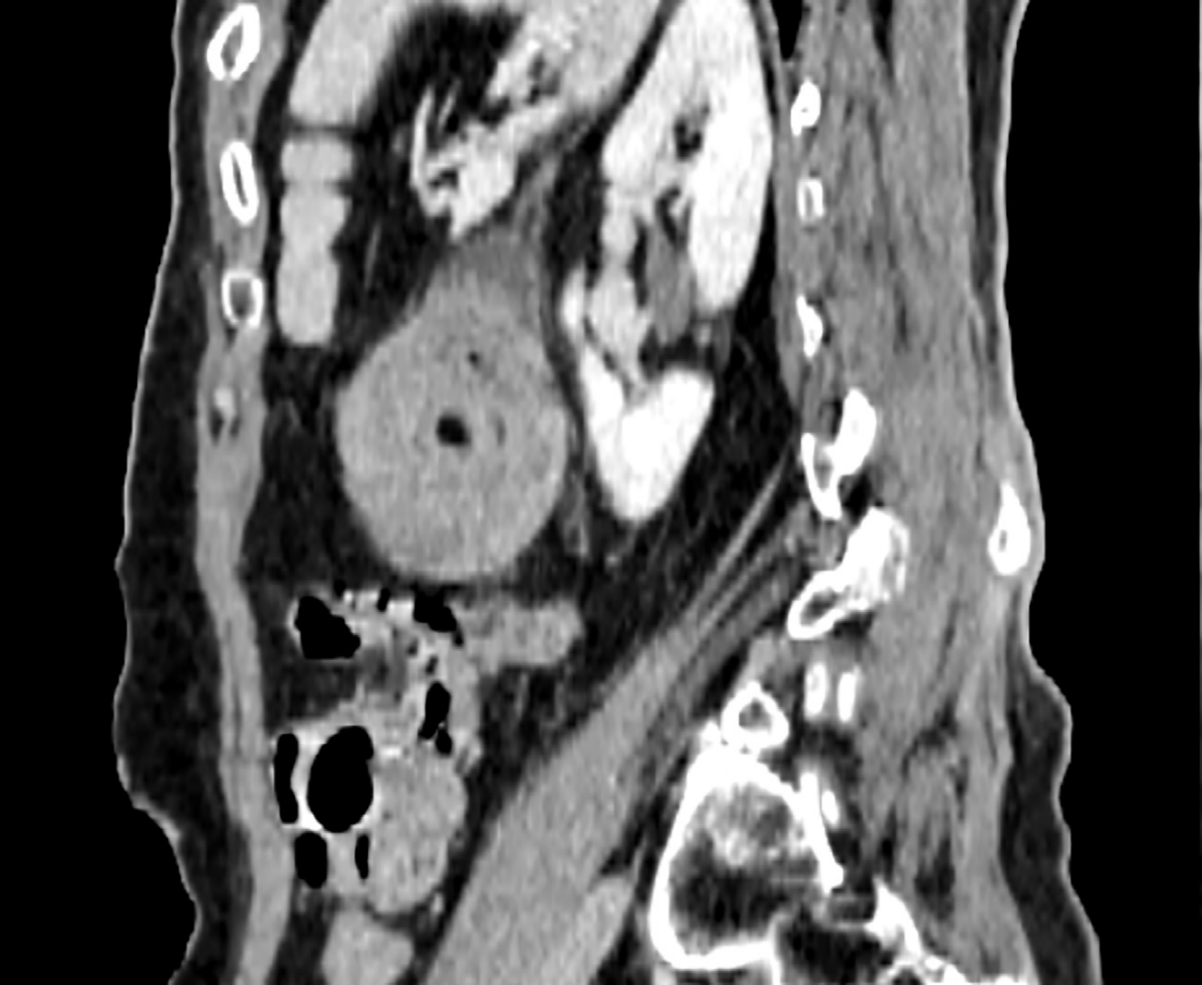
Sagittal oblique contrast-enhanced CT image showing a classic *target sign* (red arrow) corresponding to the intussusception. The concentric bowel wall layers and central mesenteric fat are clearly visualized, with the section aligned along the long axis of the invagination.
